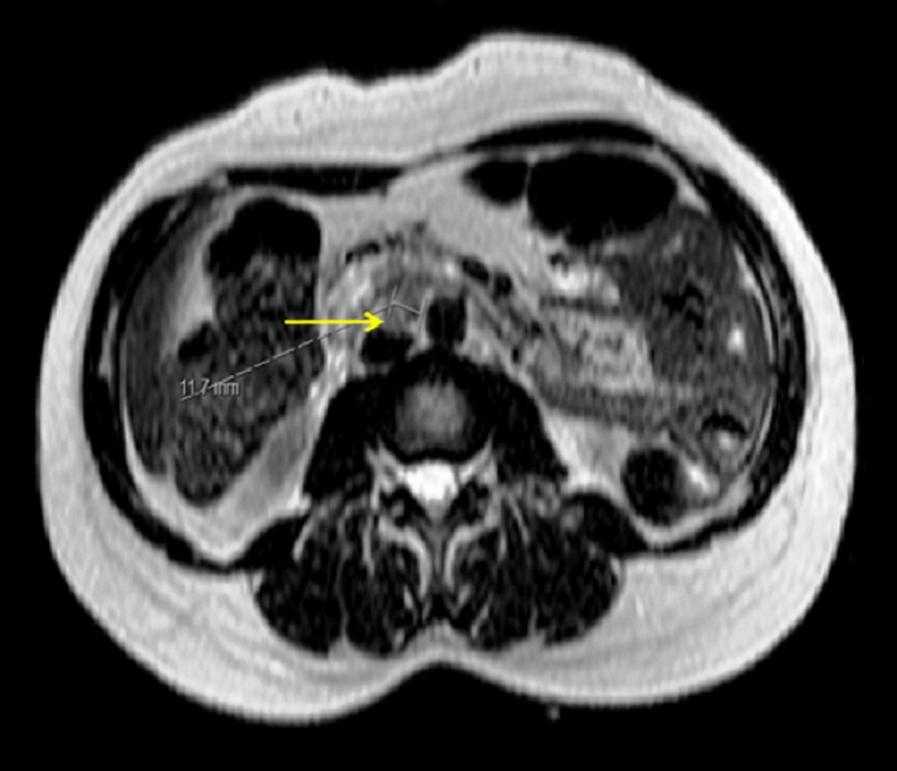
MRI abdomen On MRI abdomen, retroperitoneal and para-aortic lymph nodes appeared enlarged up to 1.3 centimeters. A 1.2-cm para-aortic lymph node was also seen just inferior to the duodenum. No mesenteric lymphadenopathy was present and there was no focal lesion in the pancreas or liver.
